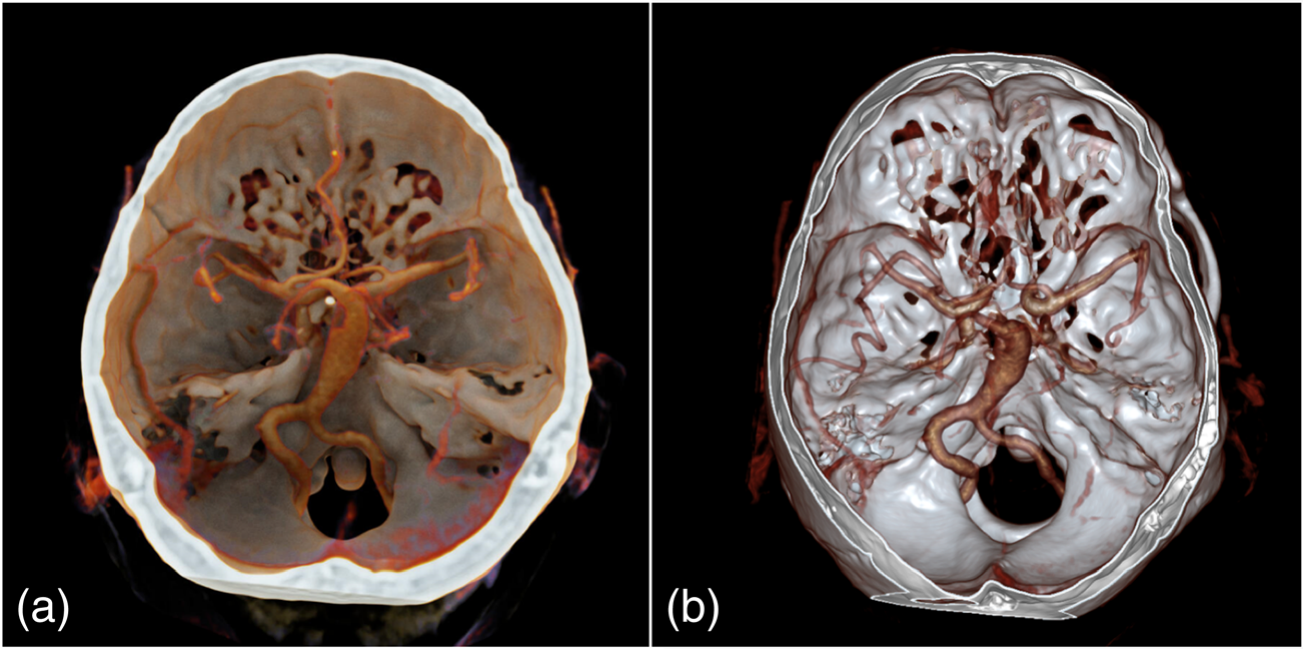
Contrast-enhanced 3-D CT images of a basilar artery aneurysm. CR (a) and conventional VR (b) depict the base of the skull with the circle of Willis, as well as the fusiform dilation of the basilar artery. Note the more natural representation of the bones and vessels in the CR image
